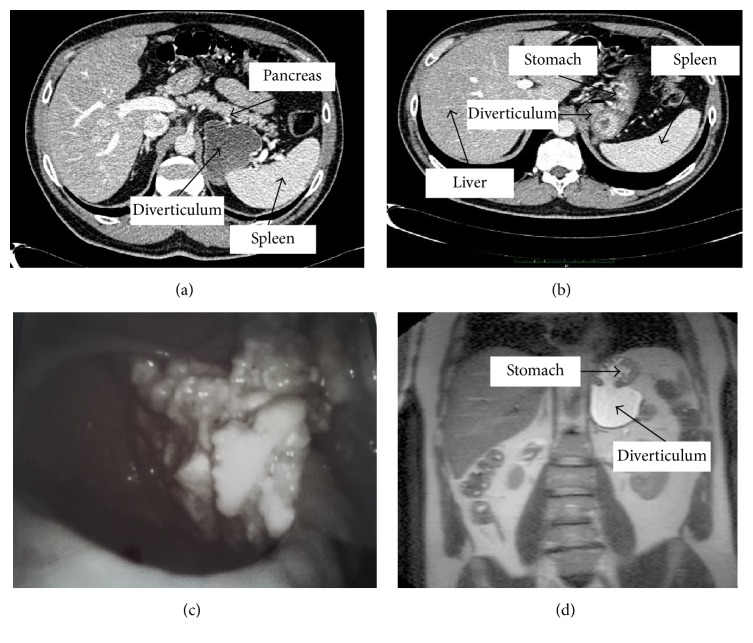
Arterial phase CT scan, transverse planes (a-b). Endoscopic image of a large diverticular neck (30 × 20 millimeters) situated in the posterior wall of the gastric fundus, with the pouch partially filled by undigested food (c). T2-weighted MRI image (d).
